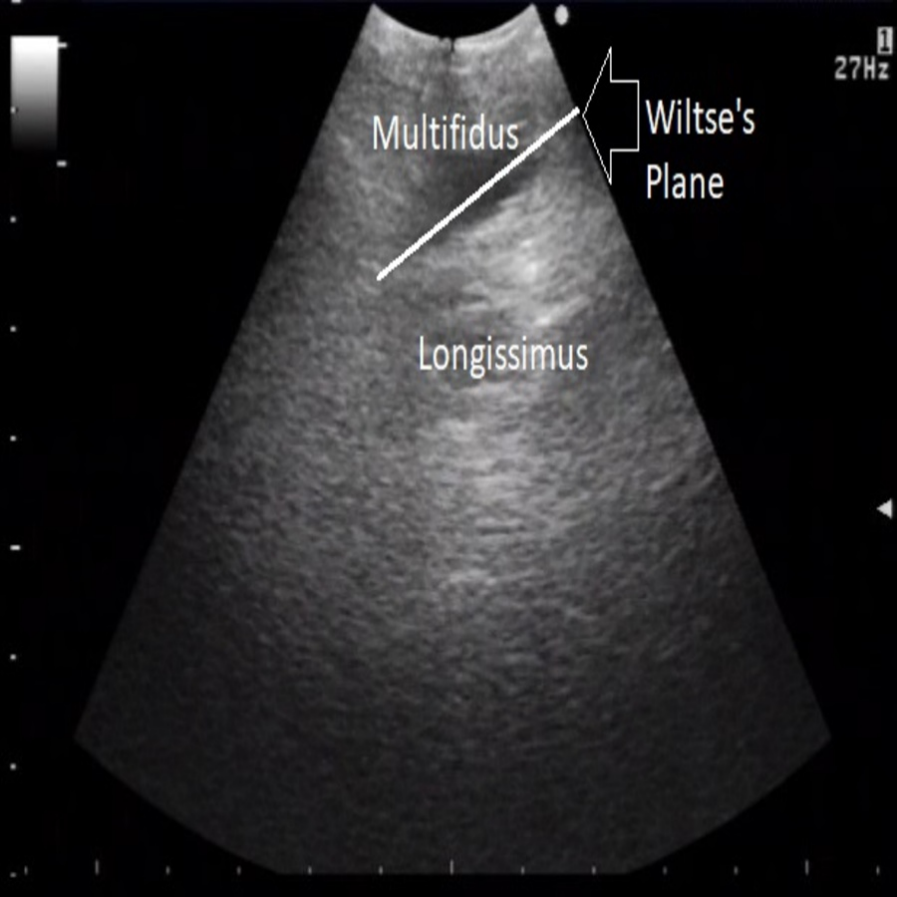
Axial ultrasound imaging: post-hydrodissection. The white line reveals Wiltse’s plane, and separates the multifidus muscle medially from the longissimus muscle laterally.
